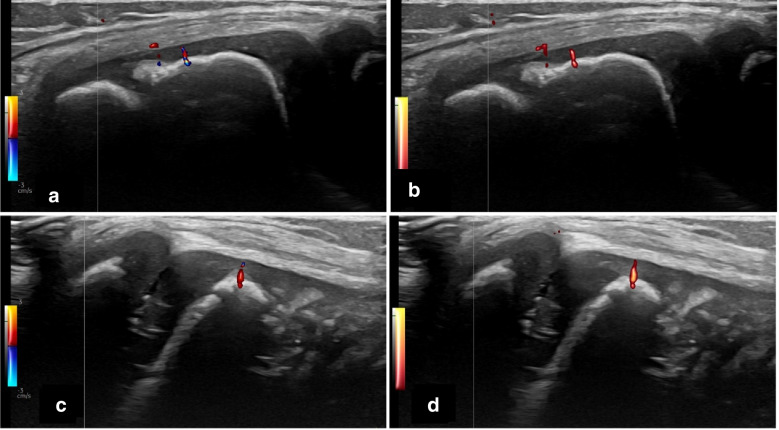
Transverse knee-ultrasound images in two 7-year-old healthy girls, at the level of the lateral parapatellar recess (a, b) and at the level of the lateral joint space (c, d). The colour Doppler (a, c) and power Doppler (b, d) images show identical findings of normal vessels in cartilage
